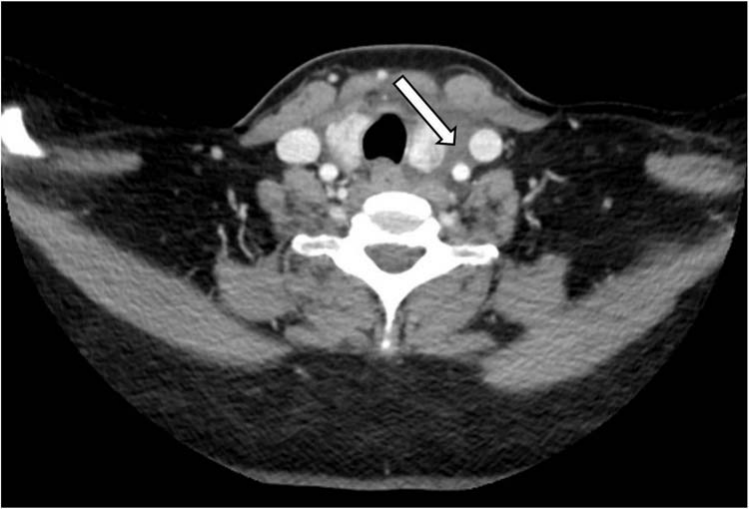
Axial section of CT-Neck showing eccentric, anteromedial inflammation surrounding the carotid arteries (white arrow).
